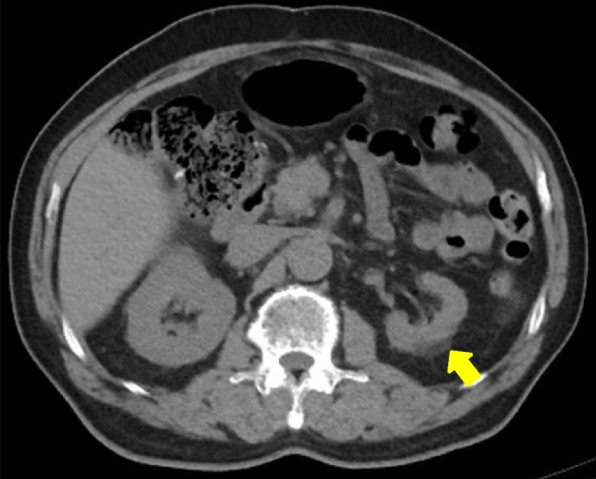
Abdominal computed tomography showed that the scar formation was detected in the area of renal infarction, on 3 months after the operation
